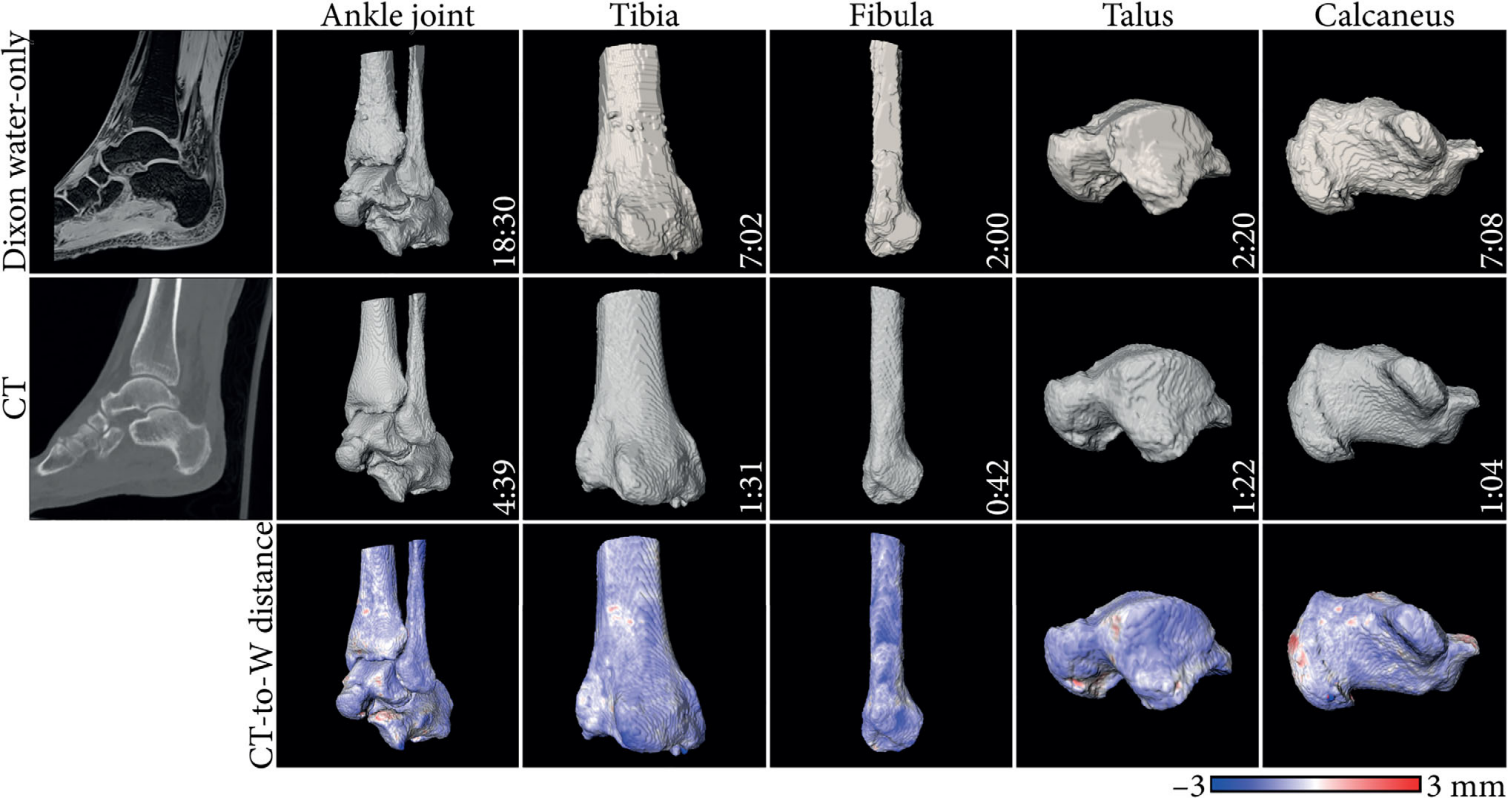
Example segmentations obtained from bone Dixon reconstructed water‐only (W) and computed tomography (CT) images of the ankle joint. Segmentations were performed by a junior engineer using Slicer 4.11 (https://www.slicer.org/). The time required to perform the segmentation is reported in the format mm:ss. Surface distance maps from CT to magnetic resonance imaging (MRI)‐based bone renderings are displayed. Negative values indicate the CT‐based segmentation is larger.
